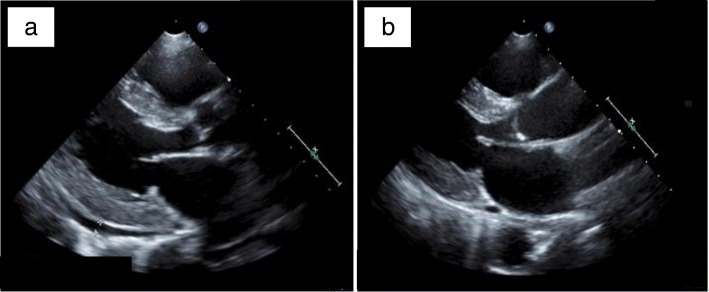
Pretreatment transthoracic echocardiogram showing diastolic left ventricular dysfunction (*E*/*e*′ = 19.2) with a well-preserved ejection fraction (73%), along with left ventricular wall hypertrophy (end-diastolic intraventricular septal thickness, 13 mm) and pericardial effusion (a). After the treatment, the diastolic left ventricular dysfunction (*E*/*e*′ = 11.7), left ventricular wall hypertrophy (IVSth = 11 mm), and pericardial effusion improved (b)
